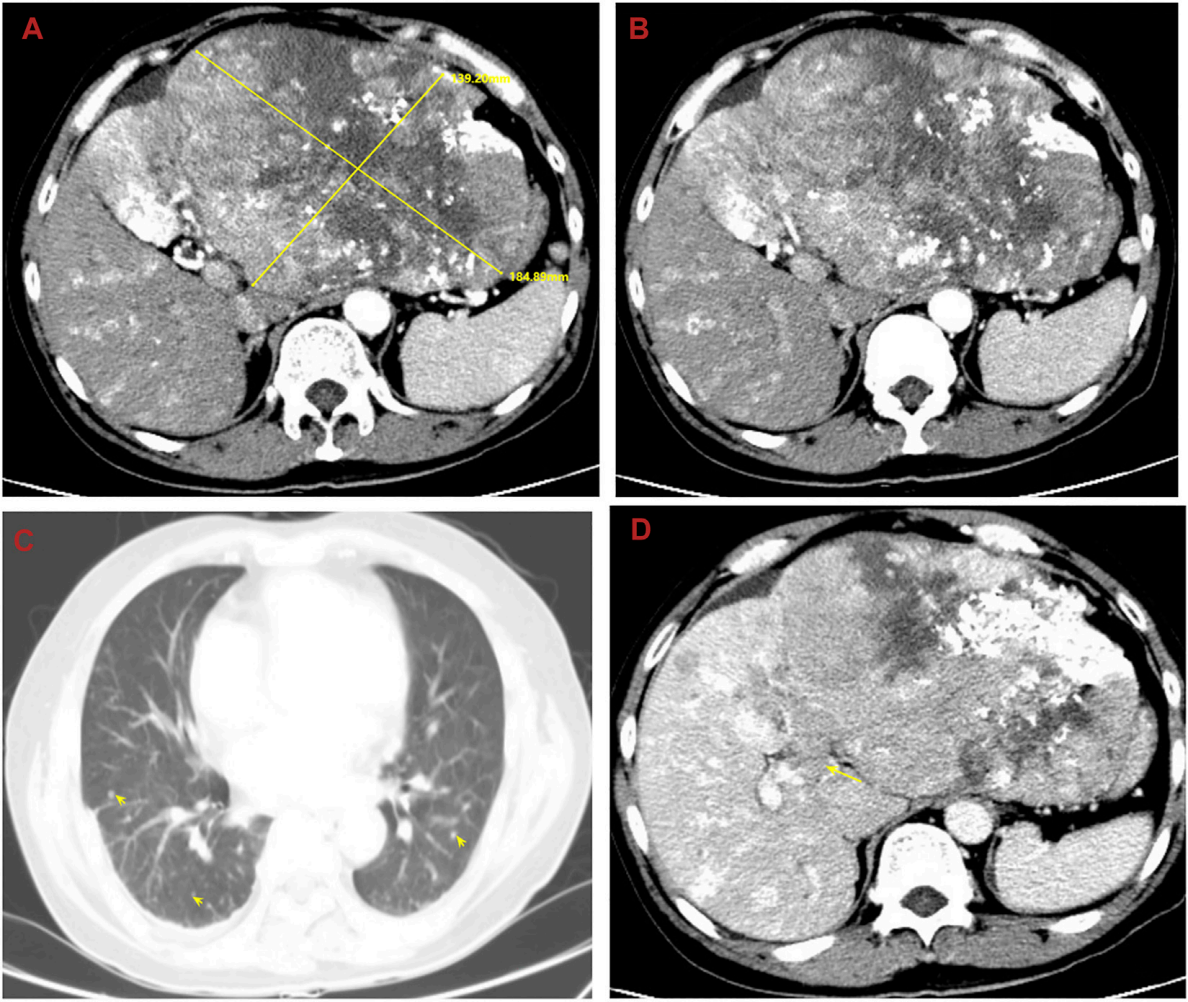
Abdominal computed tomography (CT) shows the tumor in the left lobe of the liver considered to be HCC with multiple metastases in the right lobe of the liver, and with tumor thrombosis in the left portal vein and lung metastases. (A) The huge tumor in the left lobe of the liver; (B) the multiple metastases in the right lobe of the liver; (C) the lung metastases; (D) the tumor thrombosis in the left portal vein.
